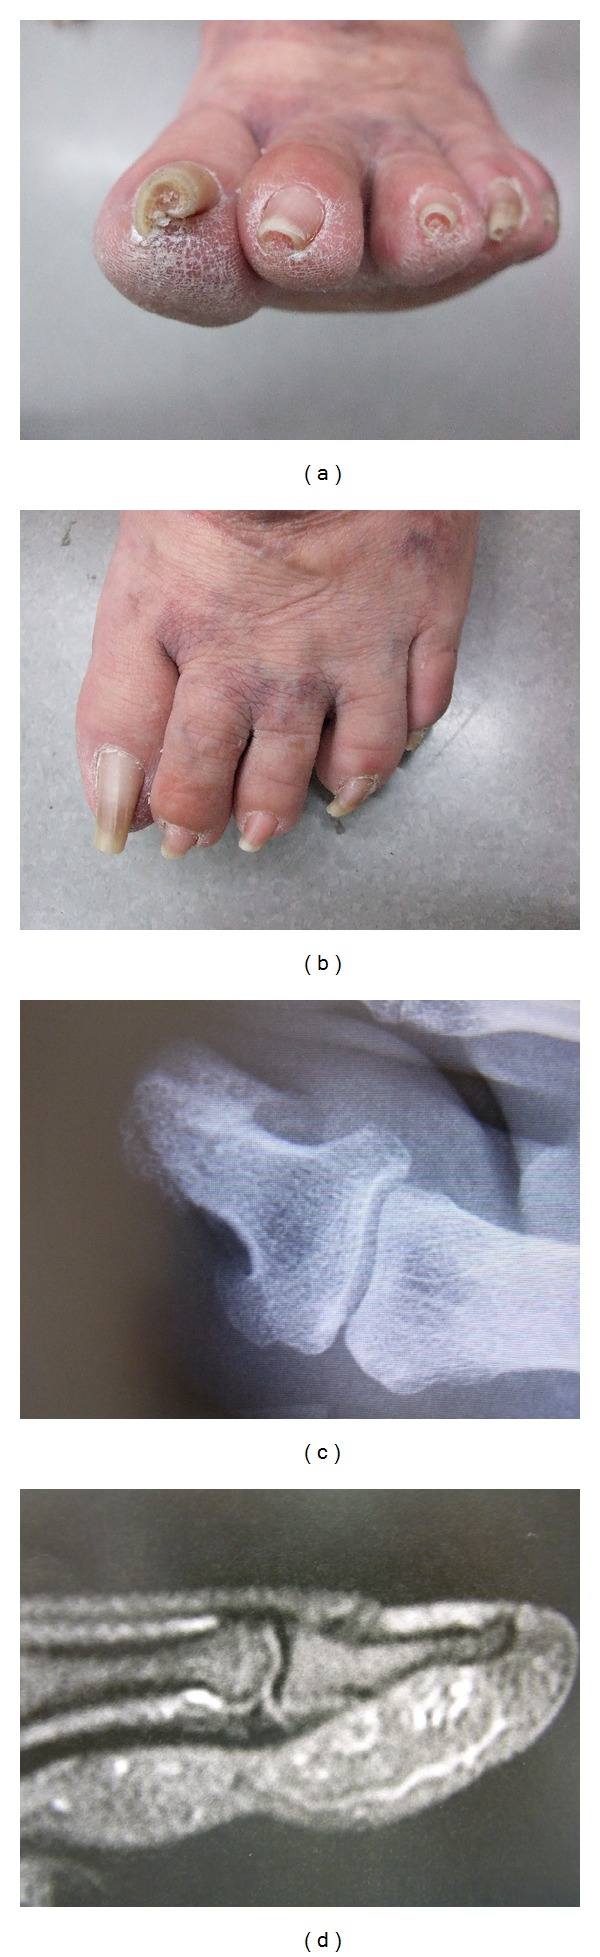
Pincer nails in a 56-year-old female patient. (a) Frontal view. (b) Dorsal view. (c) X-ray dorsal view of the distal phalanges shows the lateral deviation of the terminal phalanges and the medial hook-like exostoses at the base of the bone. (d) X-ray lateral view demonstrates the distal dorsal traction osteophyte.
